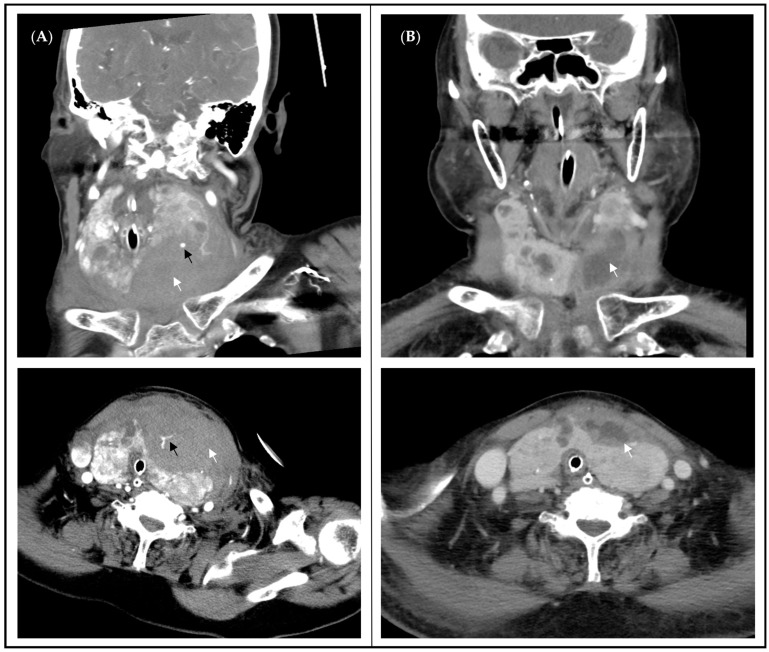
A 98-year-old woman developed hematoma after FNAC. (A): The neck CT showed enlargement of bilateral thyroid glands with multiple nodules, active bleeding (black arrow), and hematoma (white arrow), causing upper airway and left jugular vein compression with tracheal deviation. (B): The follow-up CT scan two weeks after FNAC showed hematoma (white arrow) in regression.
